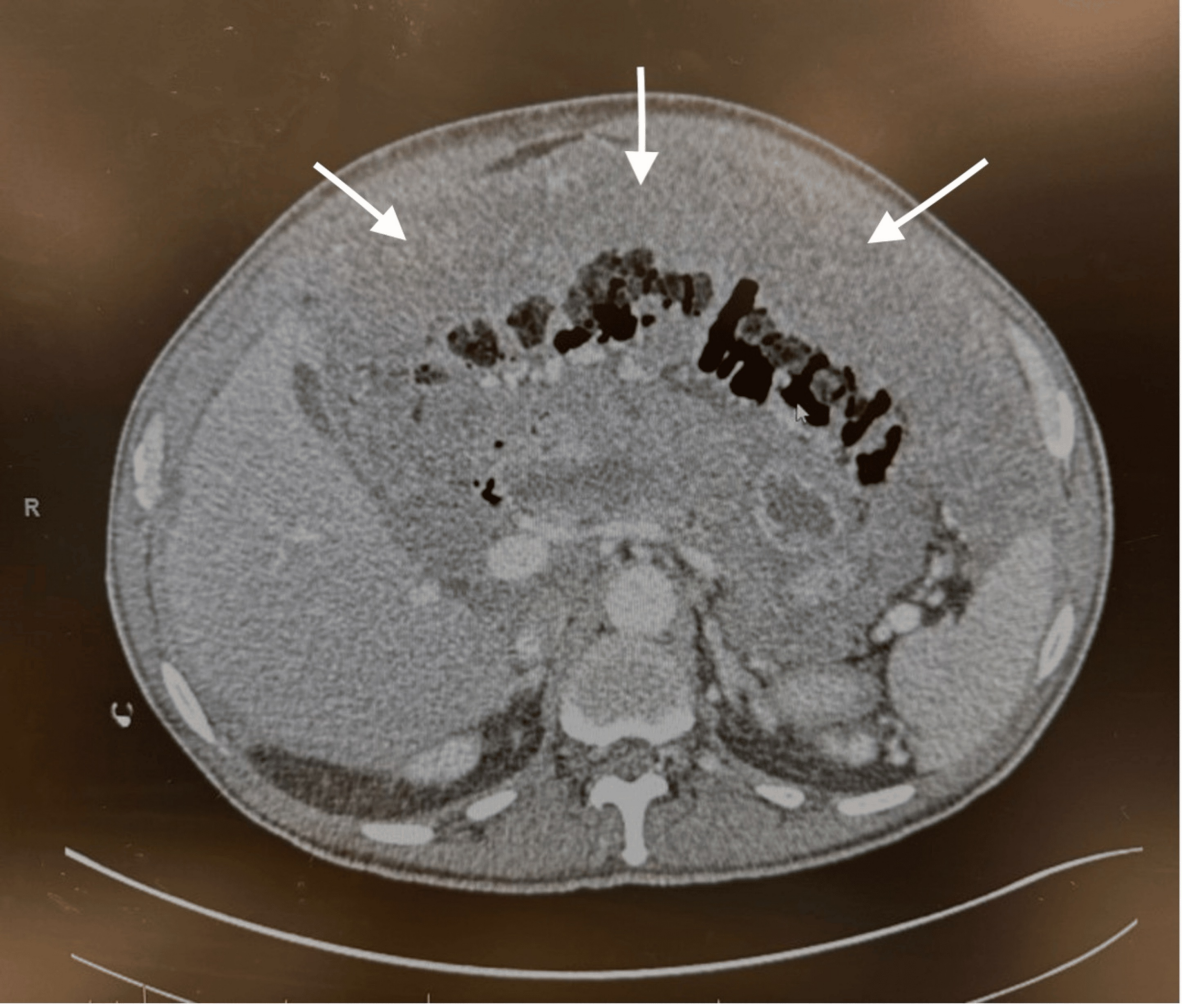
Axial CT scan shows arrows pointing to nodular implants in the abdominal peritoneum, measuring 1.4 cm and 1.8 cm
